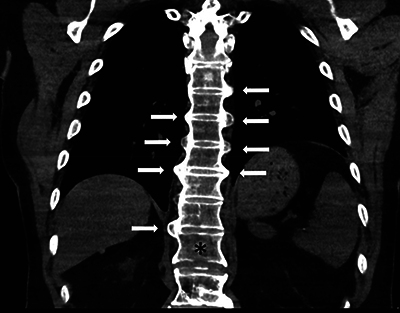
Coronal CT scan image showing the extensive syndesmophytes (arrows) along the thoracic spine and the fractured vertebra (black star).
